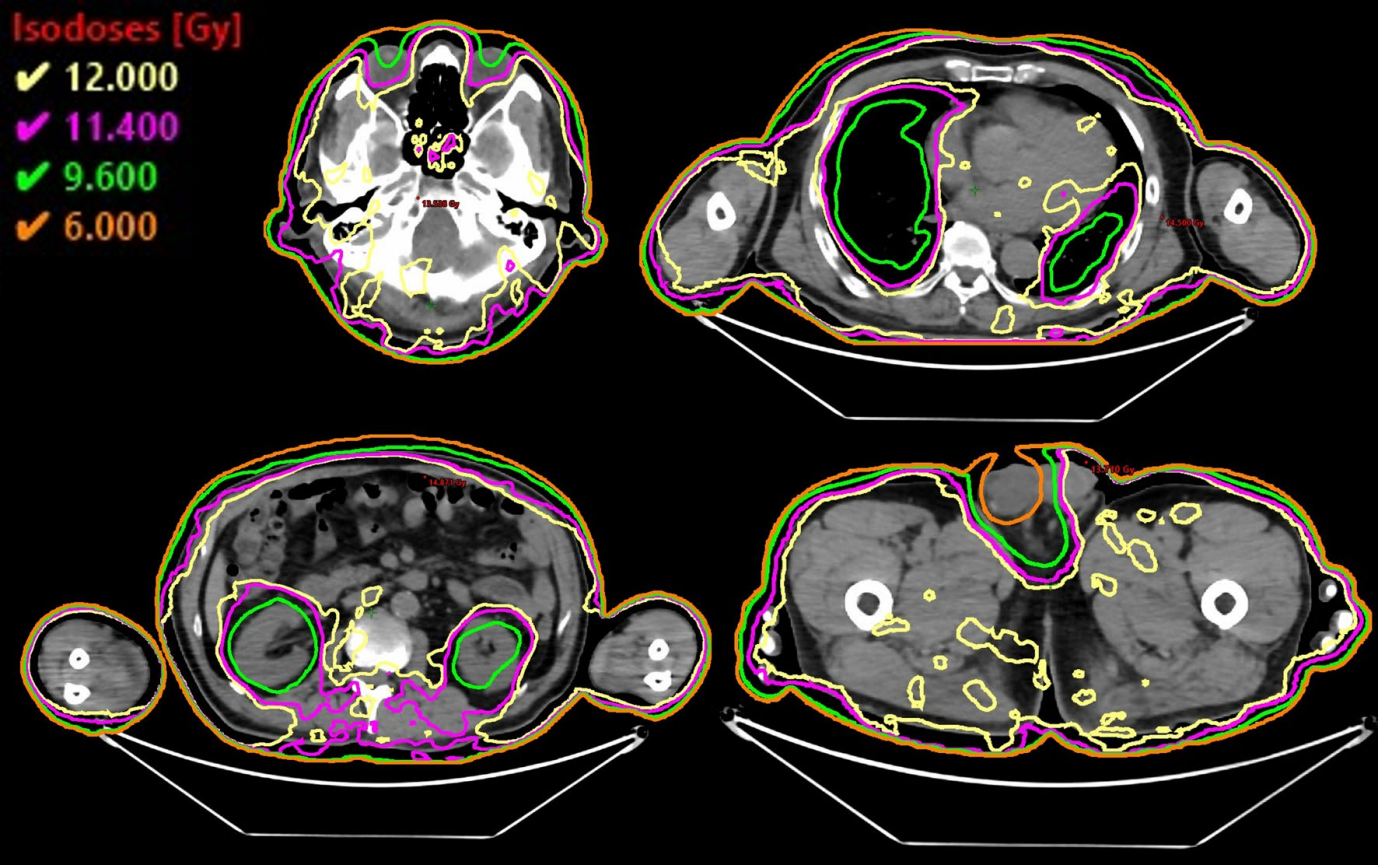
Dose distributions of different computed tomography slices, including the lens, lungs, kidneys, and testes. Yellow line, 12 Gy (100%) iso-dose line; magenta line, 11.4 Gy (80%) iso-dose line; green line, 9.6 Gy (80%) iso-dose line; orange line, 6.0 Gy (50%) iso-dose line
